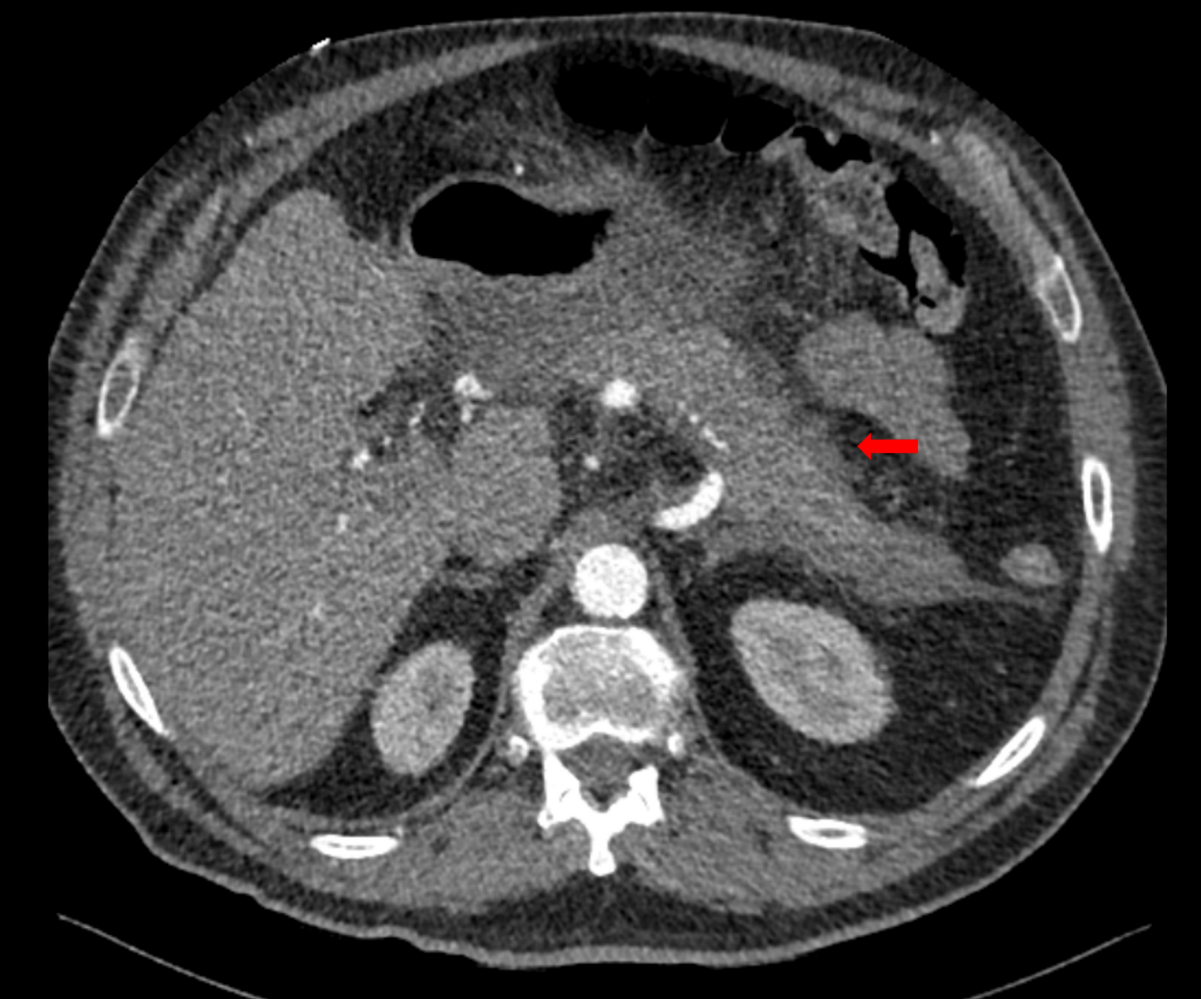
Computed tomography angiogram of the abdomen showing a diffusely enlarged pancreas Red arrow: diffusely enlarged pancreas with significant abnormal density in the peripancreatic region
